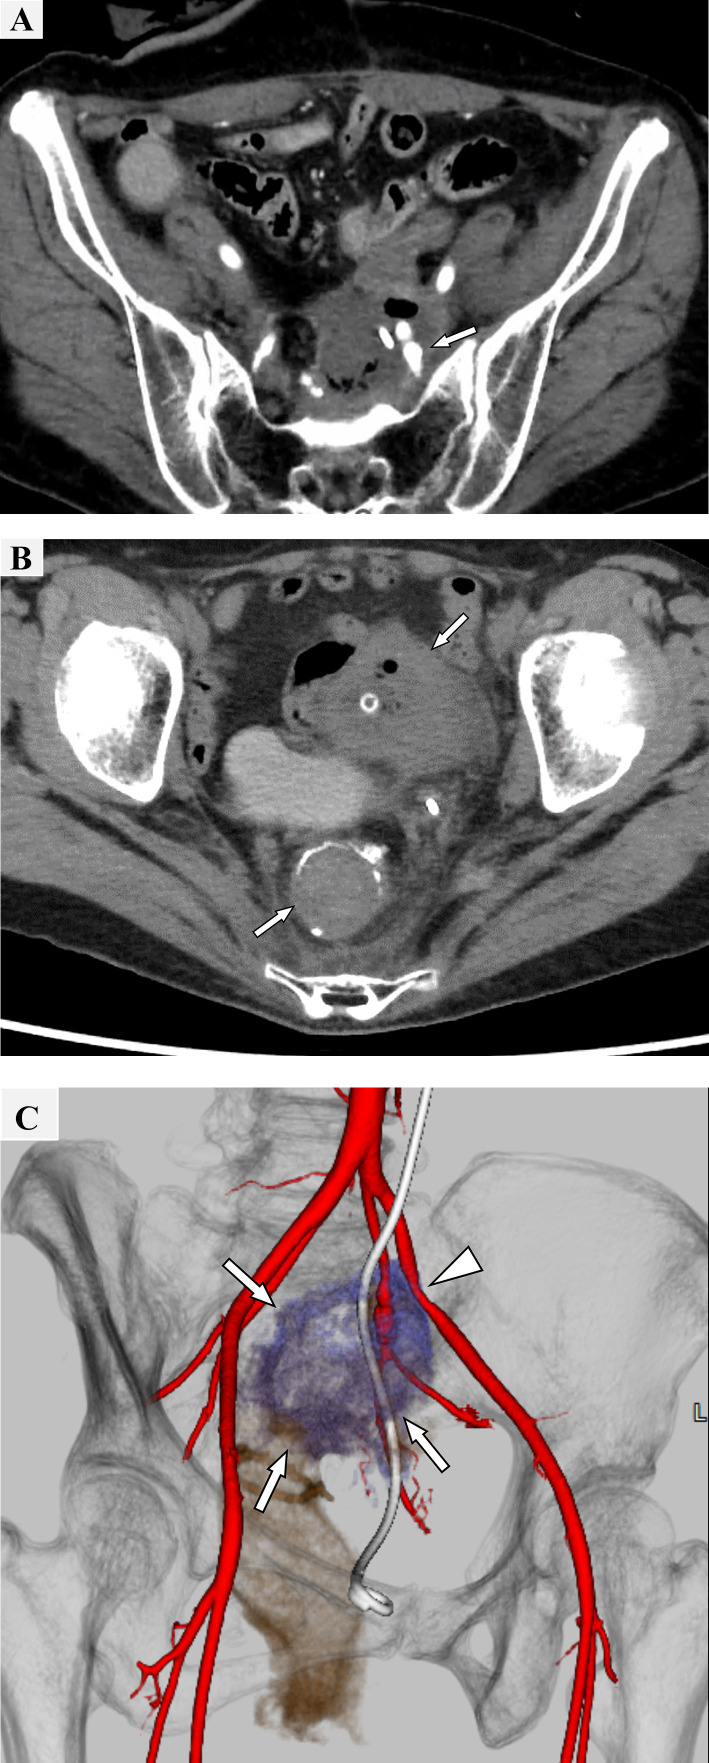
Computed tomography imaging findings of the ureterorectal-arterial fistula. (A) Contrast-enhanced Computed Tomography (CT) in the arterial phase revealed a pseudoaneurysm in the left internal iliac artery (arrow). (B) Noncontrast-enhanced CT revealed a hematoma in the bladder and rectum (arrow). (C) Volume rendering reconstruction revealed that the tumor invaded the left ureter, rectum, and left internal iliac artery (arrow). Pseudoaneurysm formation was detected in left internal iliac artery. Left external iliac artery stenosis was also observed (arrowhead).
